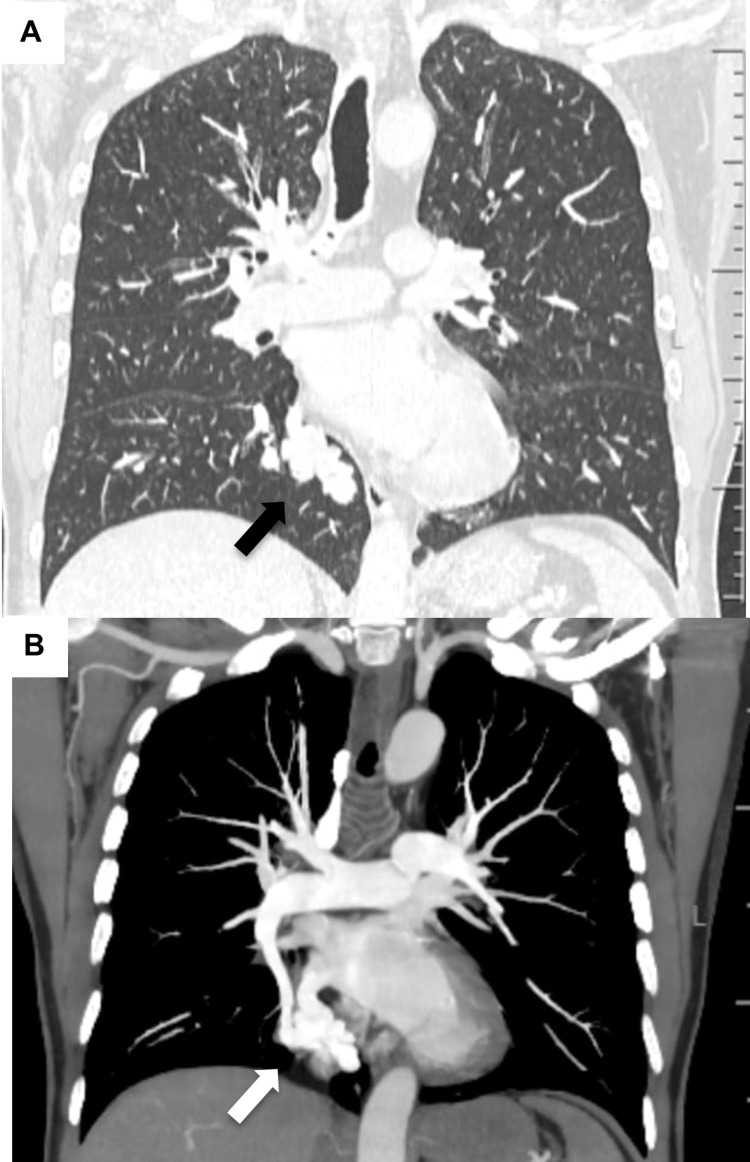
Computed tomography angiography of the chest (A. lung window, B. mediastinal window) depicting the arteriovenous malformation in the right lower lobe (arrows).
